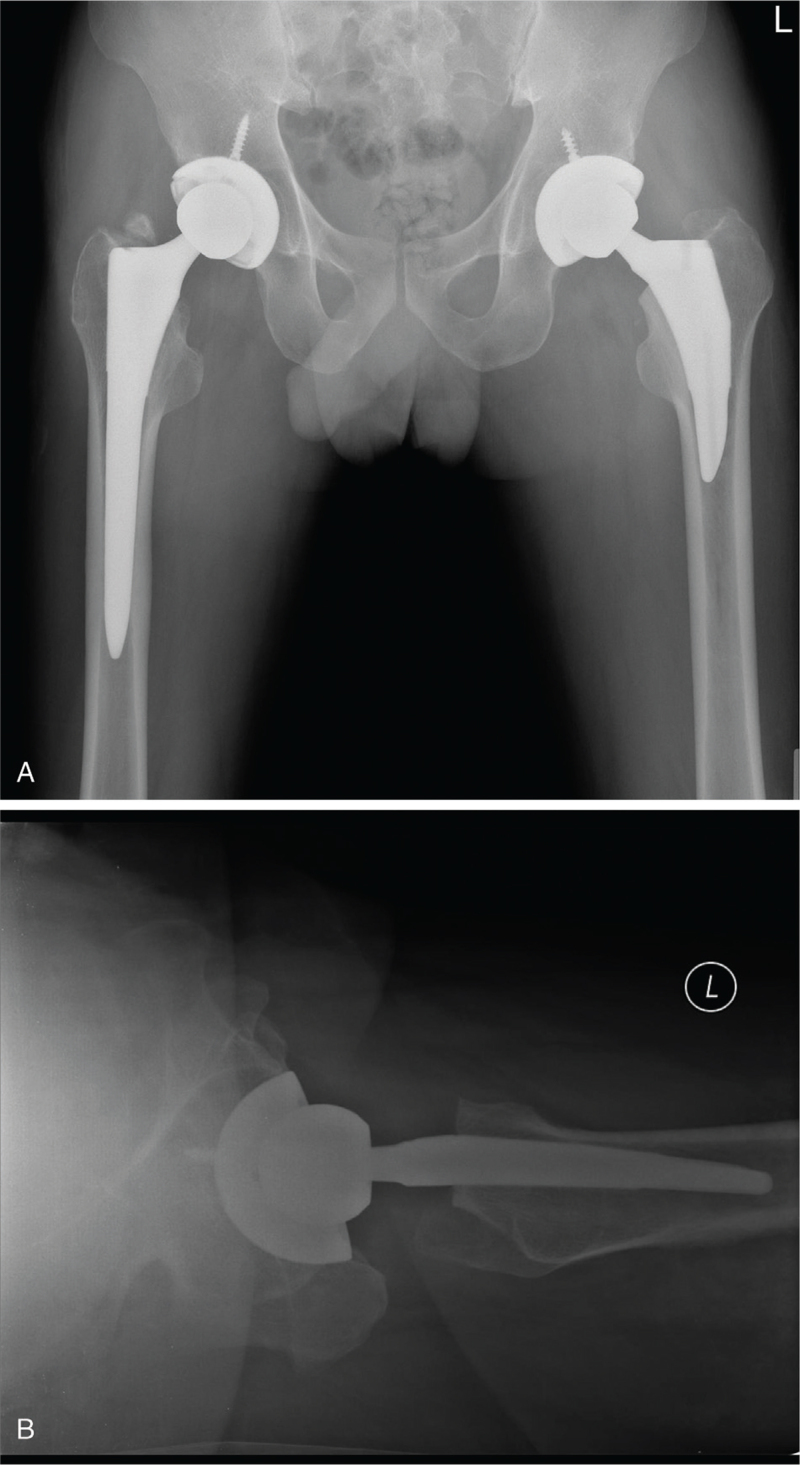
(A) Plain anteroposterior (AP) radiograph (B) cross-table lateral radiograph taken 6 weeks post-surgery showing acceptable position of the components.
